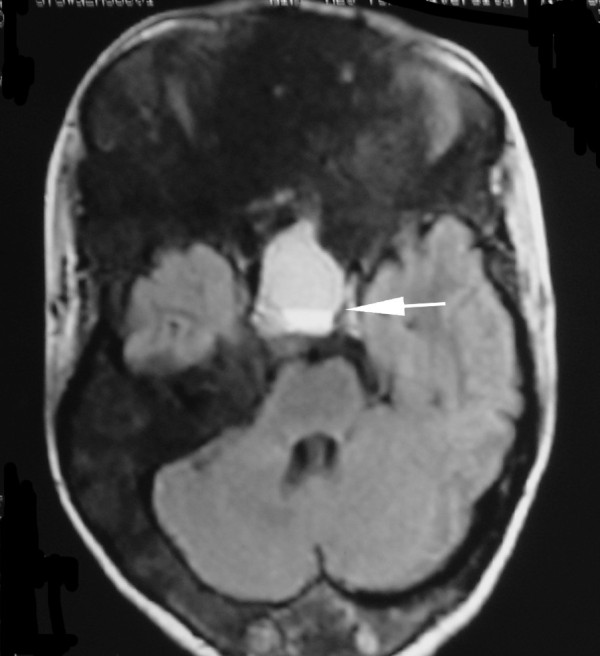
Fluid-fluid level can be showed clearly on FLAIR T1-weighted images (arrow).
